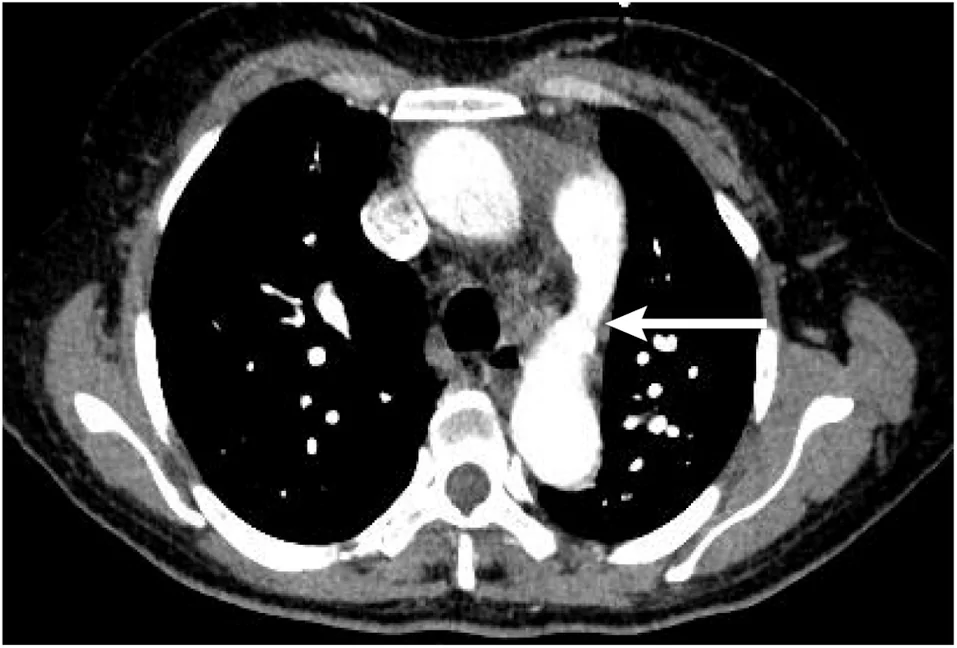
Enhanced CT demonstrated significantly enlarged patent ductus arteriosus connected the left pulmonary artery and the descending aorta (arrow).
